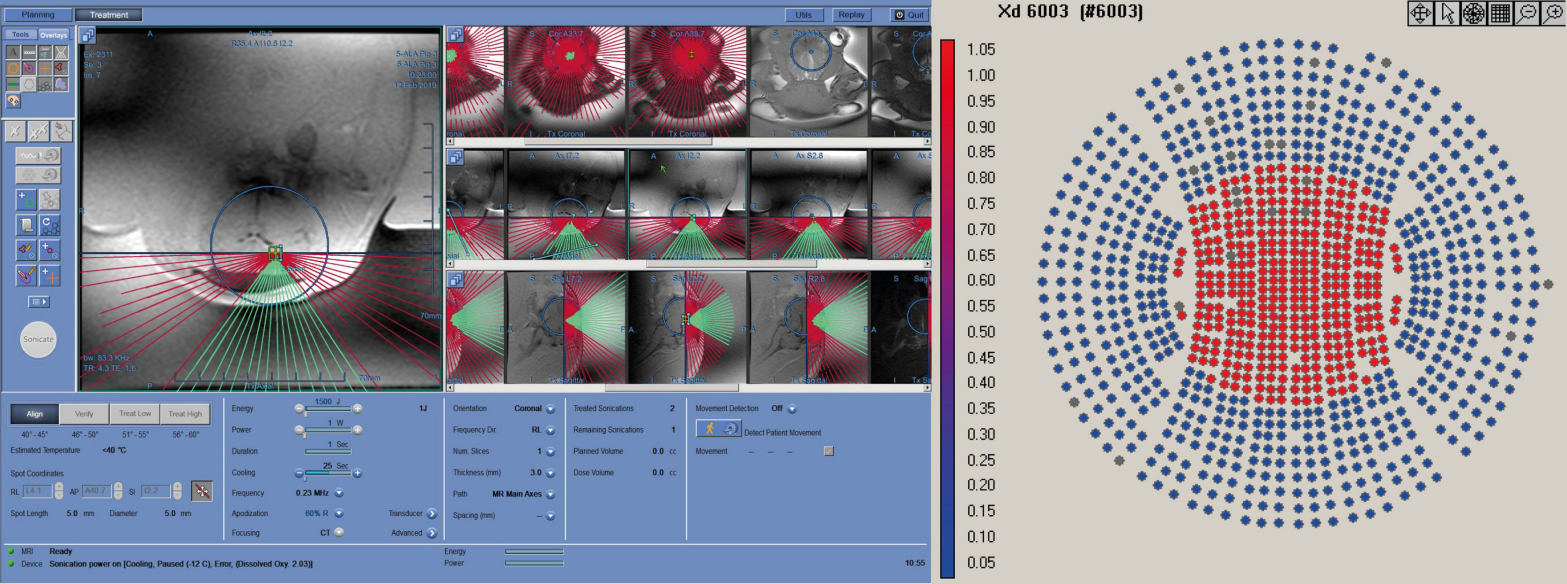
T1- and T2-weighted images acquired after the sonication procedure.
